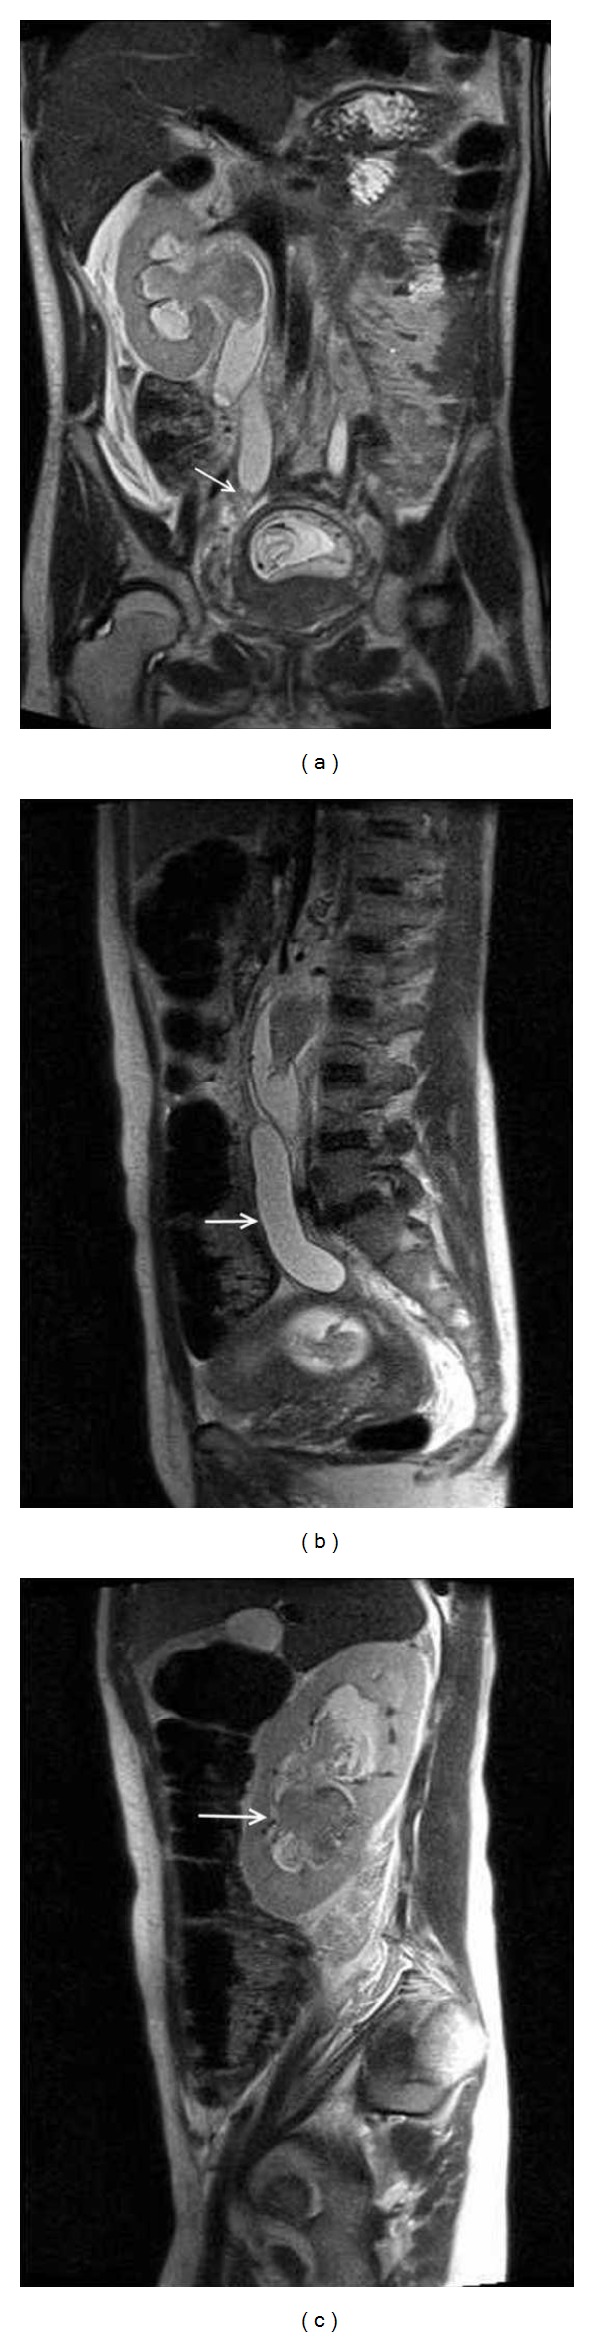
(a) MRI (coronal view) demonstrating high-grade stenosis of right distal ureter (arrow). Gravid uterus is also seen. (b) Sagittal view of tortuous left hydroureter (arrow). (c) Sagittal view of left hydronephrosis with blood (arrow) in the collecting system.
